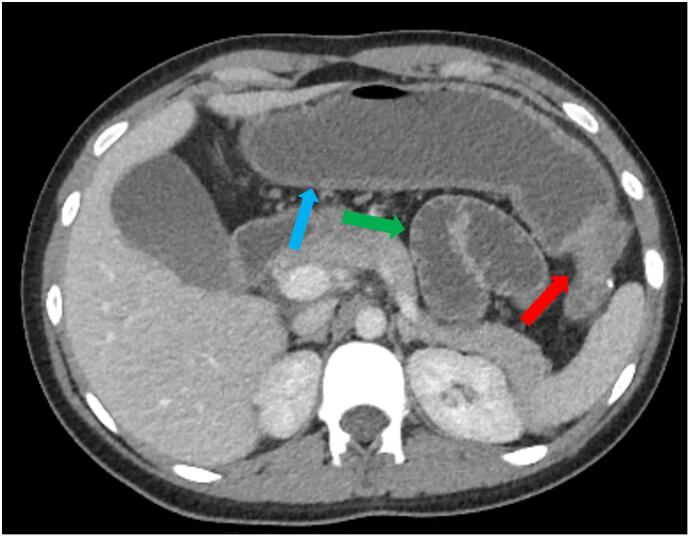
Axial CT of the abdomen and pelvis showing distended transverse colon with wall thickening (blue arrow), focal narrowing at the splenic flexure (red arrow) with distended small bowel (green arrow).
